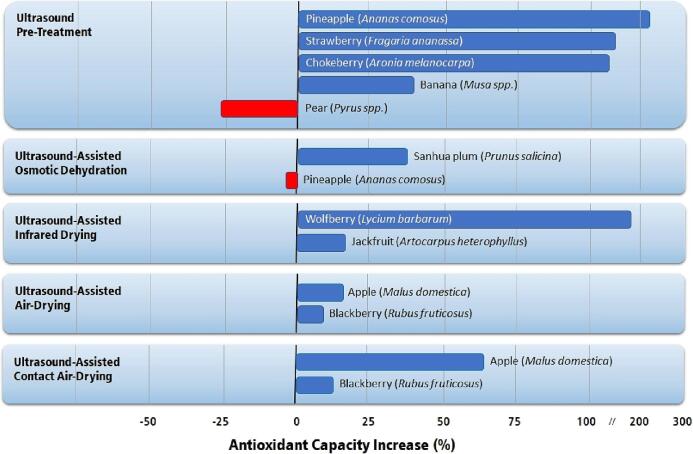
Highest increase in antioxidant capacity in fruits by applying ultrasound technologies compared to conventional air-drying [24], [38], [39], [92], [95], [98], [99], [100], [102], [104], [119].
